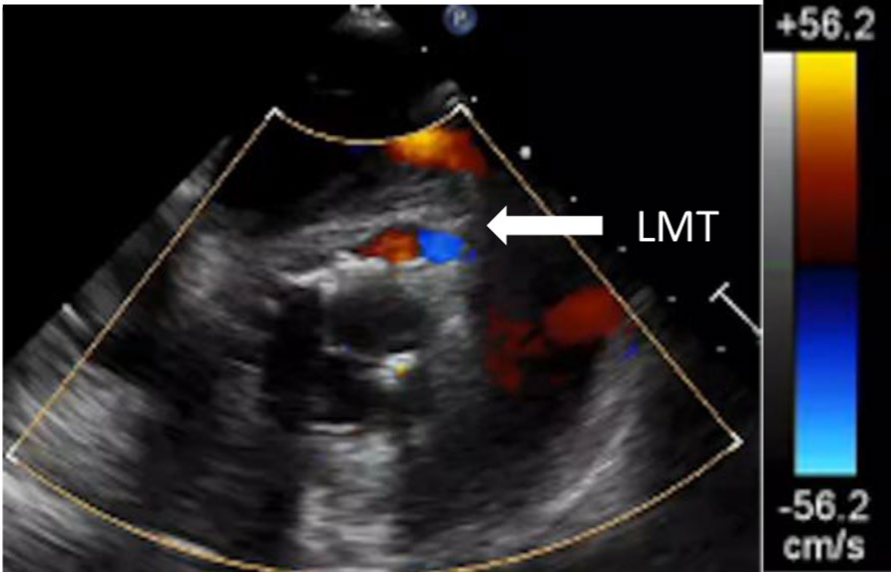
Transesophageal echocardiogram findings just after de-clump. More than 56.2 cm/s flow was detected in the left main trunk (LMT)
